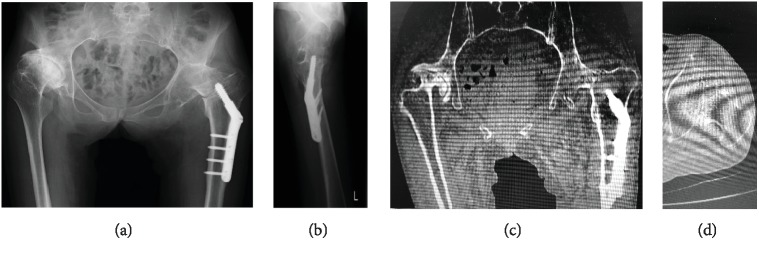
The plain radiograph and CT of both hip joints of a 64-year-old woman. Left hip joint showed the femoral neck fracture after postoperative intertrochanteric fracture in the fused hip. Right hip joint shows severe osteoarthritis due to DDH. (a) Anteroposterior view; (b) lateral view; (c) sagittal view of CT image; (d) axial view of CT image.
